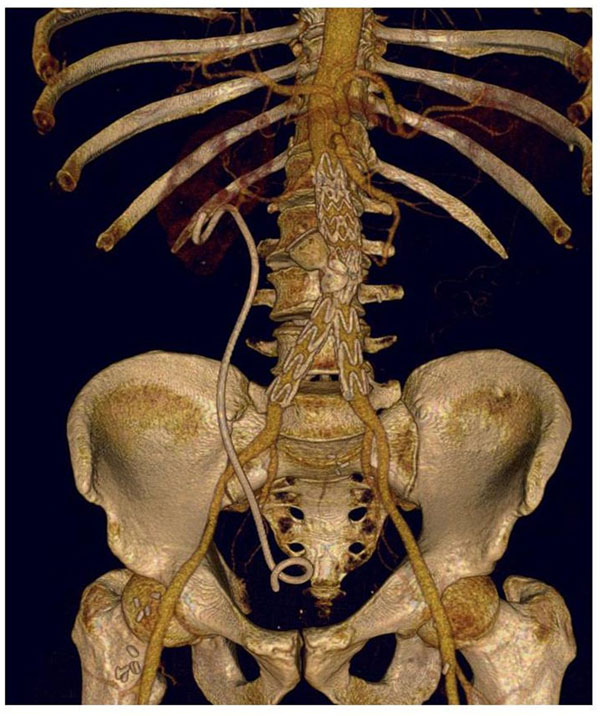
The CT scan 1 month after aortic endoprosthesis implantation showed a complete abolishment of the shunt with no opacization of the inferior vena cava from the abdominal aorta . The Amplatzer vascular plug was excluded from the aortic blood flow after the endoprosthesis implantation.
